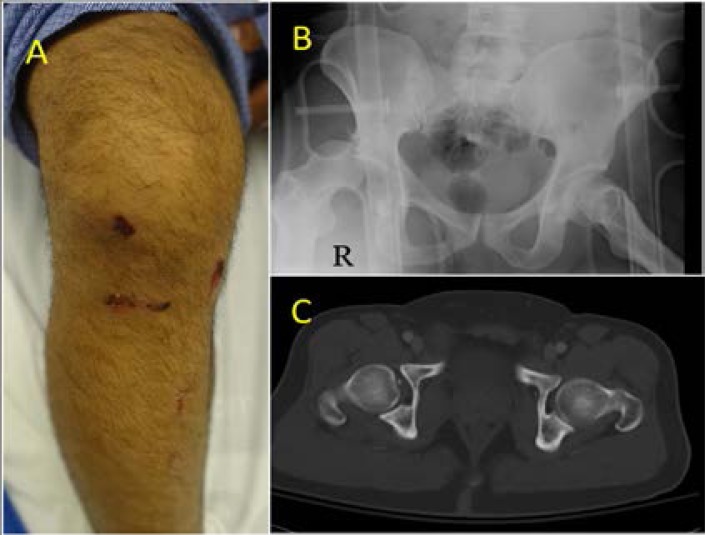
42-year-old male driver had a frontal impact collision presented complaining of the right knee and hip pain. The right hip was internally rotated with flexion (60 degree). The right knee was swollen having multiple abrasions (A). Pelvic X-ray showed posterior dislocation of the right hip (B). Post reduction CT scan showed fracture of posterior rim of the acetabulum and widened hip joint space (C).
